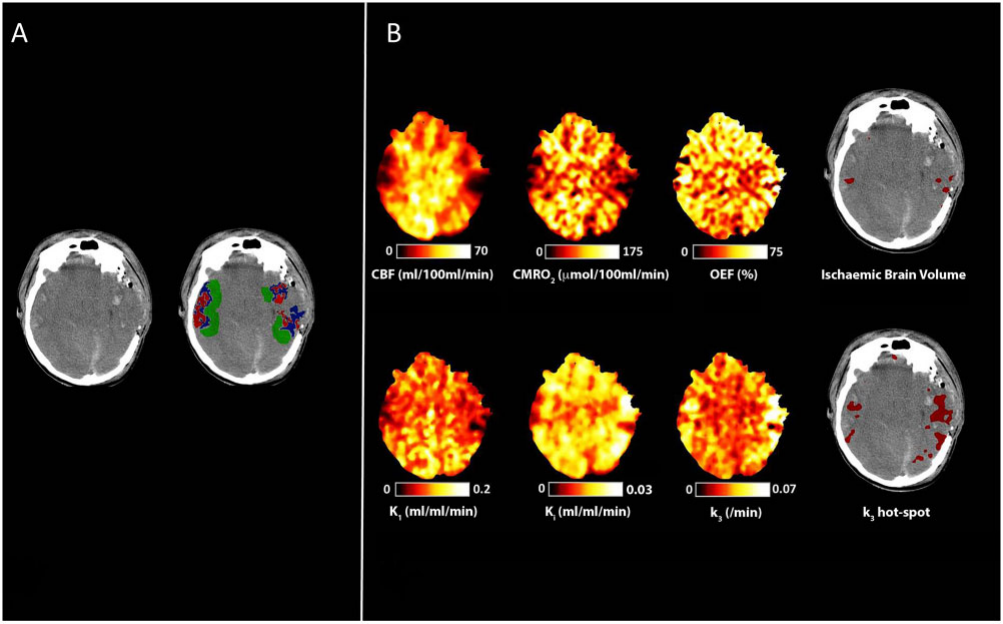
Peri-lesional hyperglycolysis in the absence of cerebral ischaemia. CT, CBF, CMRO_2_, OEF, and ^18^F-FDG kinetic parameters obtained in a 29-year-old male within 24 h of severe TBI following a road traffic accident. Plasma glucose during imaging was 6.6 mmol/l. (A) CT demonstrates bilateral temporal and parietal haemorrhagic contusions. Regions of interest are defined for haemorrhagic lesion (core, red), hypodense tissue (penumbra, blue), and a 1 cm border zone of normal-appearing tissue (peri-penumbra, green). (B) Co-registered parametric maps of CBF, CMRO_2_, OEF, K_1_, K_i_ and k_3_. The regions with a critical increase in OEF above the individually calculated threshold (ischaemic brain volume) and region of increased phosphorylation activity (k_3_ hotspot) are both shown in red overlying the CT scan. The ischaemic brain and k_3_ hotspot volumes were 7 ml and 56 ml, respectively, and overlap between these two tissue classes in this patient was 0.4 ml.
